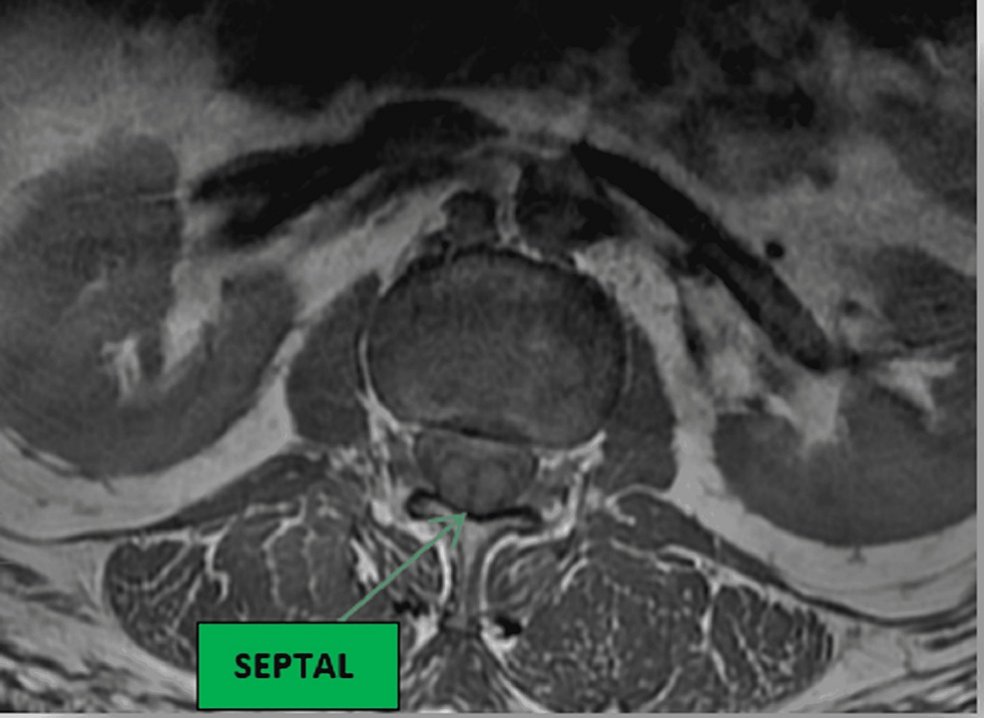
T1-weighted axial lumbar MRI showing a split cord septal at the level of L2-L3.
